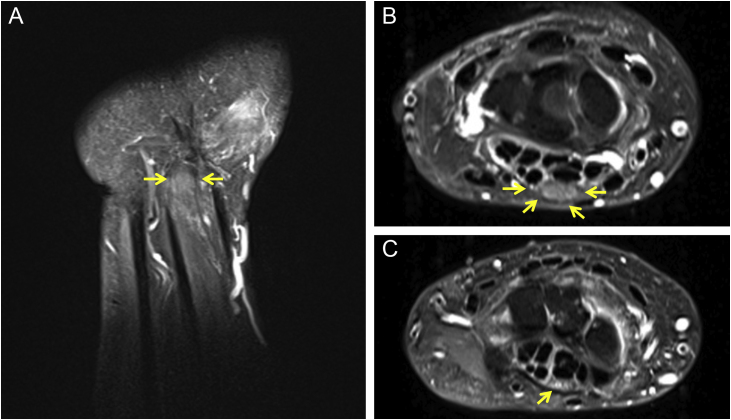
Magnetic resonance imaging of the right wrist. (A) Sagittal view showing thickening of the median nerve (arrows). (B) Axial view showing thickening of the median nerve in the carpal tunnel (arrows). (C) Nerve showing normal thickness outside the carpal tunnel (arrow).
